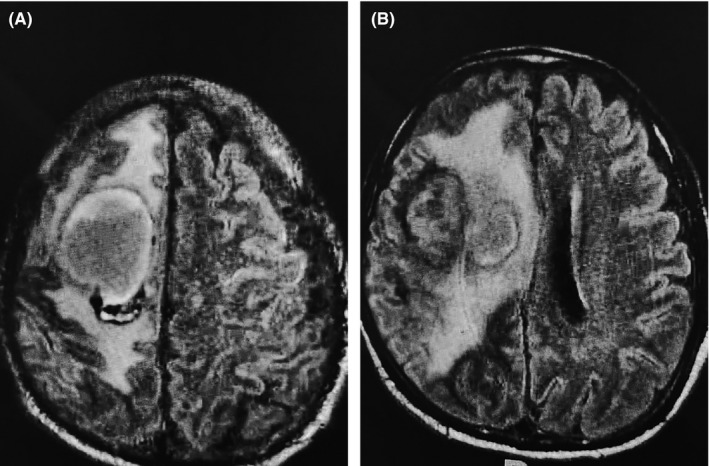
A, B MRI T2 Flair images
